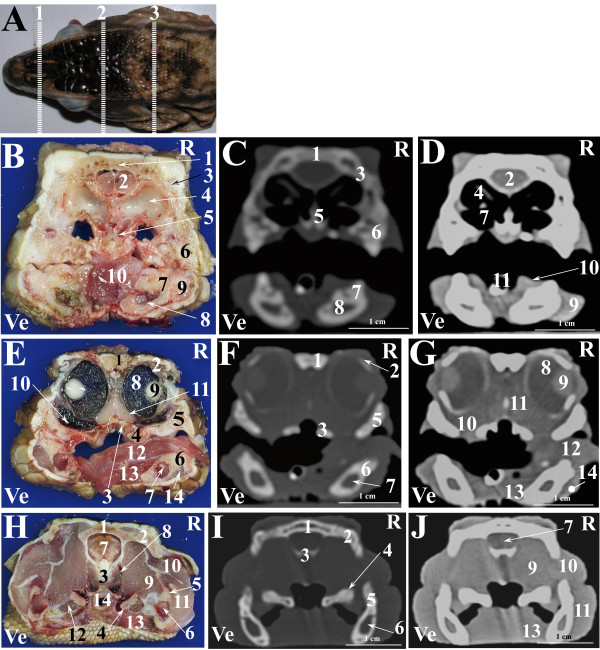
Normal computed tomographic anatomy of the head of the common tegu.A. Photograph of the head of a tegu; lines (1–3) indicate the approximate levels of matched cross-sections and CT images. B- J: Matched images of cross-sectional gross anatomy ( B- E- H), CT image displayed in bone window (WW: +2000; WL: +500)( C- F- I) and CT image displayed in soft tissue window (WW: +400; WL: +40) ( D- G- J) corresponding to: line 1 ( B- C- D), line 2 ( E- F- G) and line 3 ( H- I- J) as depicted in Figure 6A. R is right and Ve is ventral. B-C-D: 1. Nasal bone; 2. Nasal glands 3. Prefrontal bone; 4. Nasal conchae; 5. Septomaxilla; 6. Maxillary bone; 7. Dentary bone; 8. Meckelian canal 9. Infralabial glands; 10. Tongue; 11. Trachea. E- F- G: 1. Frontal bone; 2. Scleral ossicles; 3. Palatine bone; 4. Palatine gland; 5. Maxillary bone; 6. Dentary bone; 7. Meckelian canal; 8. Eye; 9. Lens; 10. Sinus orbitalis; 11. Harderian gland; 12. Tongue; 13. Musculus intermandibularis + Musculus geniohyoideus + Musculus genioglossus + Musculus hyoglossus; 14. Infralabial glands. H- I- J: 1. Parietal bone; 2. Squamosal bone; 3. Sphenoid bone; 4. Pterygoid bone; 5. Angular bone + Surangular bone; 6. Adductor fossa; 7. Brain; 8. Musculus longus colli; 9. Musculus adductor mandibulae externus profundus; 10. Musculus abductor mandibularis externus medialis; 11. Musculus intermandibularis posterior; 12. Musculus pterygoideus typicus + Musculus pterygoideus atypicus; 13. Musculus intermandibularis + Musculus geniohyoideus + Musculus pterygoideus typicus + Musculus hyoglossus; 14. Trachea.
